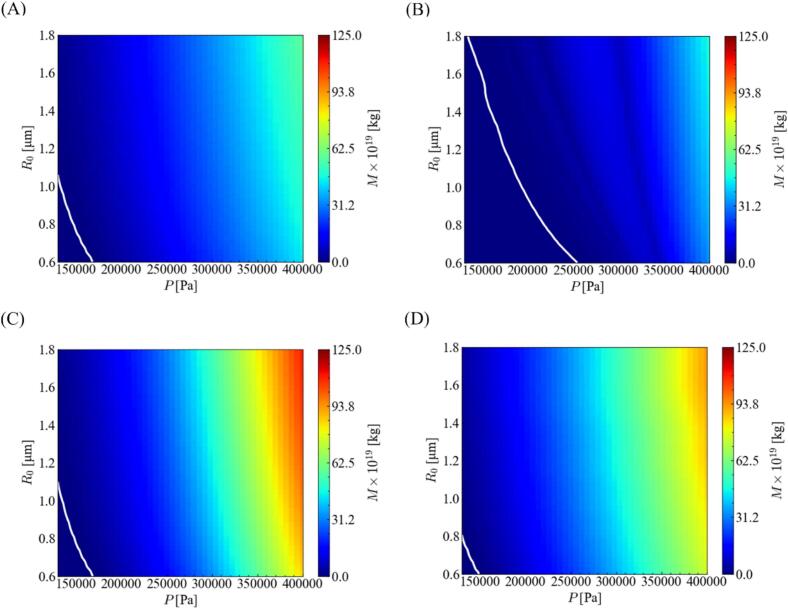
Distribution of the total mass change of the bubble in an ultrasonic cycle for the case of *α*_1_ = 0.7 with different phase shifts: *θ* = (A)-*π*, (B)-*π*/2, (C) 0, and (D) *π*/2. The region on the left from the white line indicates the total mass change of the bubble becomes negative, which indicates the condition where the gas in the bubble dissolves into the liquid and the bubble shrinks.
